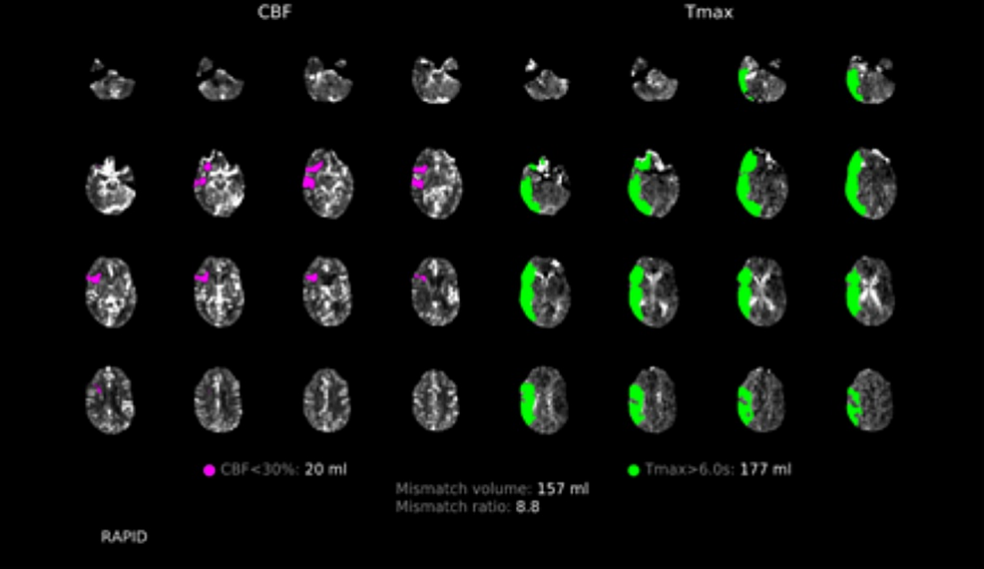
CT perfusion scan showing large penumbra:core mismatch
